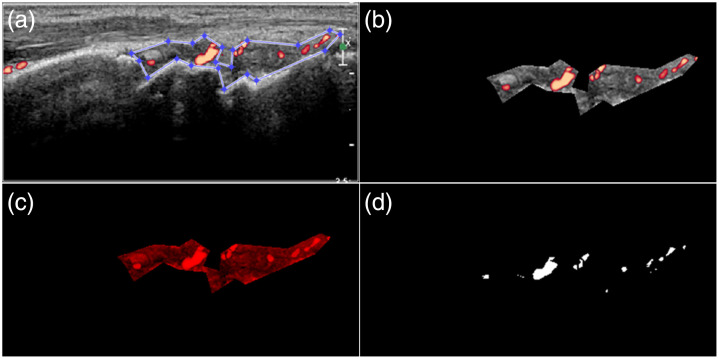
Computer-aided quantitative analysis of PD ultrasound image of the wrist. (a) Original image shows color flows in the hypertrophied synovium. The boundary of the synovium is delineated manually. (b) Segmentation of the synovium. (c) Decomposition of the RGB image and obtaining a red-gray image. (d) Convert the red-gray image to a binary image with an appropriate threshold then calculate the area of PD.
